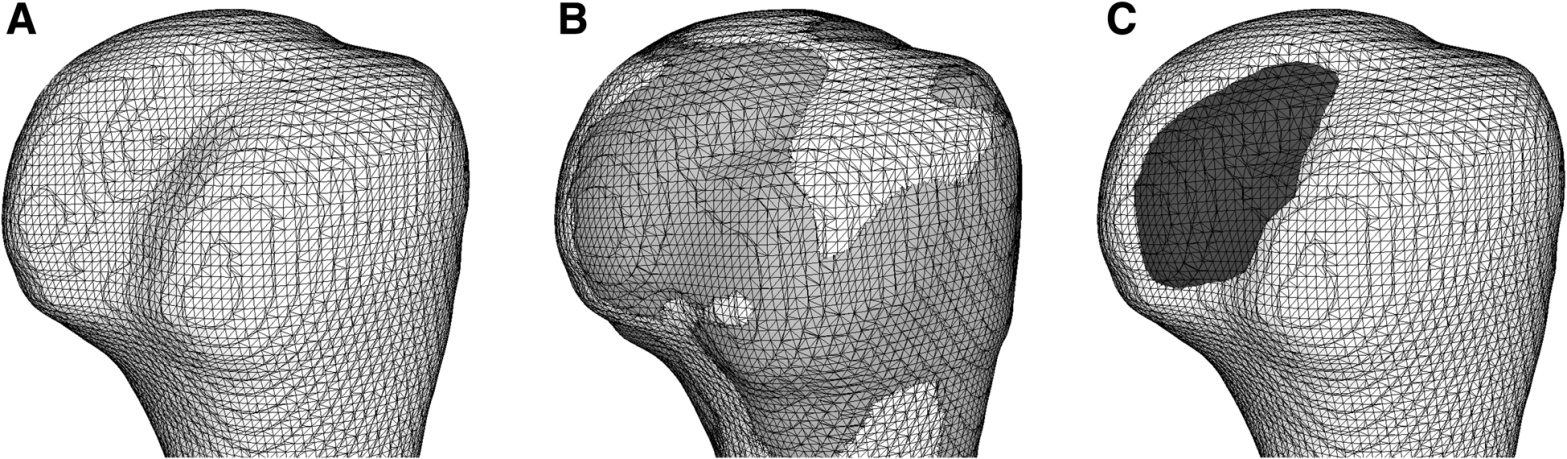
Identification of humeral head bone defects. a Three-dimensional surface model of the right proximal humerus (involved side) is reconstructed from the obtained computed tomography data. b The mirrored surface data of the left proximal humerus (intact side) is matched to the right glenoid model using the anterior half of the proximal humerus. c The bone defect area (black) is identified with subtraction of the affected-side volume from the intact side
